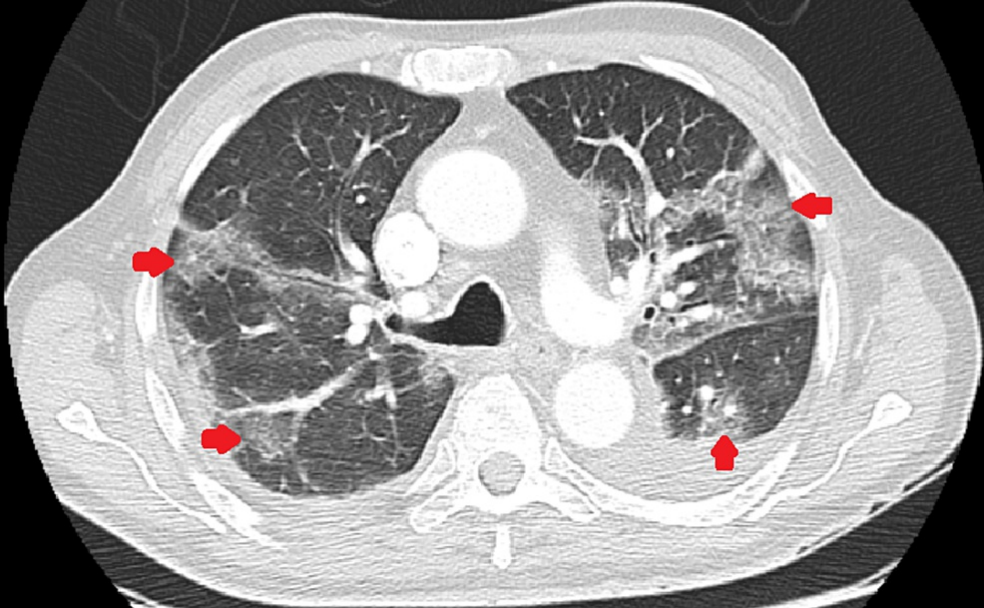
Thoracic CT angiography performed in March 2021 Red arrows: bilateral multifocal ground-glass opacities, with adjacent interstitial densification, presenting a crazy paving pattern.
